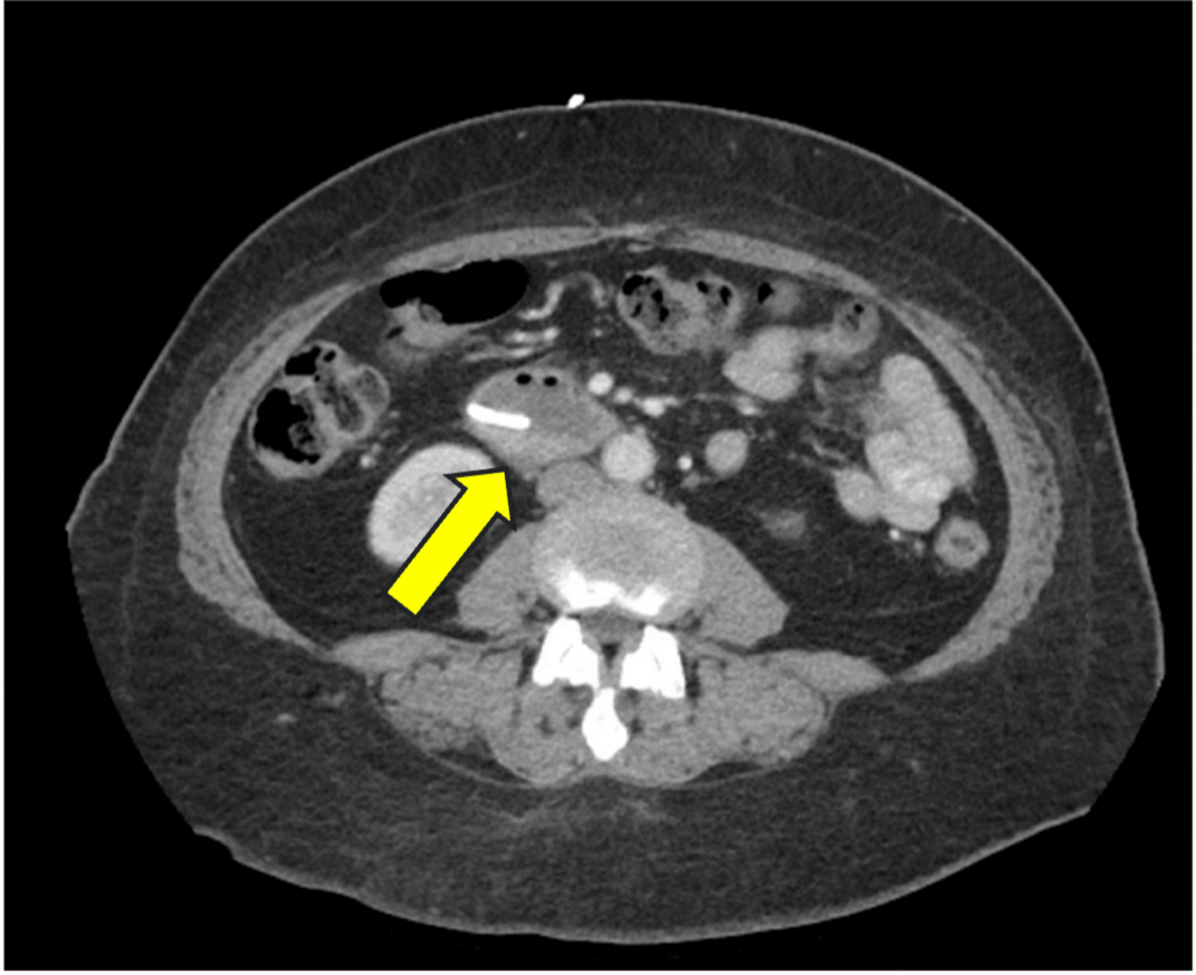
Radiographic response of the primary tumor one month after RT. Axial view with the yellow arrow pointing to the pancreaticoduodenal mass. RT, radiation therapy
